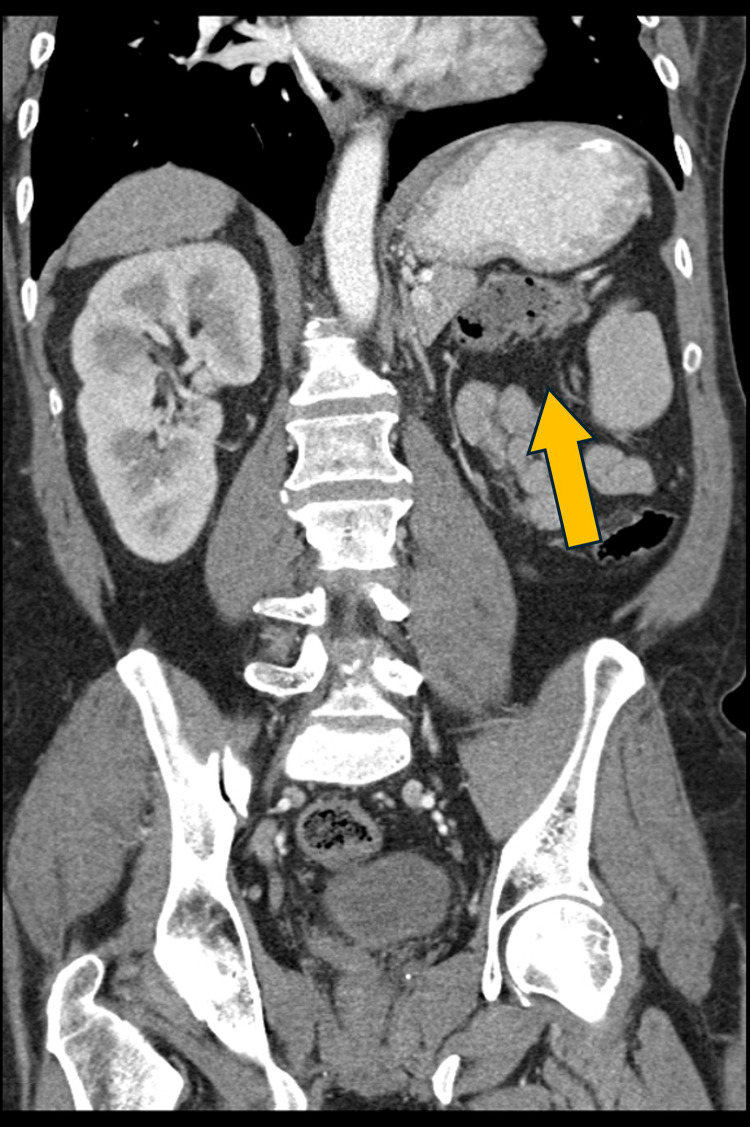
CT shows unilateral renal agenesis (yellow arrow) in this patient, which has been documented as a rare occurrence associated with KFS.
